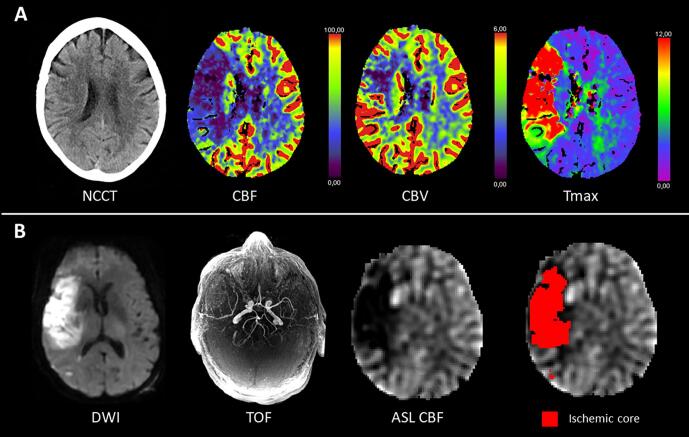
Example images of a patient presenting with a right M1-MCA occlusion and substantial neurological deficit (baseline NIHSS = 12) who was not successfully treated with thrombectomy (eTICI 0). (A) Top row includes baseline CT images showing large perfusion deficit in the right MCA territory. (B) Follow-up MR imaging at 24 h in bottom row. DWI shows a large area of infarction with a persistent occlusion of the right M1-MCA on TOF-MRA. ASL CBF map shows hypoperfusion in the ischemic core (red). This patient’s neurological deficit increased at 24 h (NIHSS = 17).
